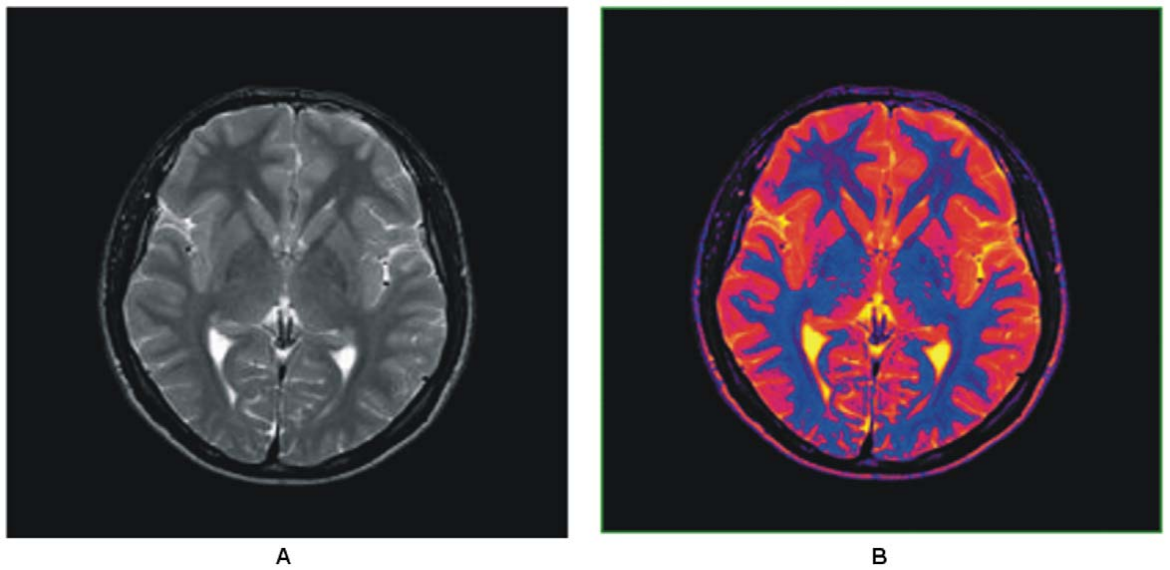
Color transformed T2 brain MR image. (A) Gray scale brain MR image. (B) Colorized brain MR image obtained using proposed method.
